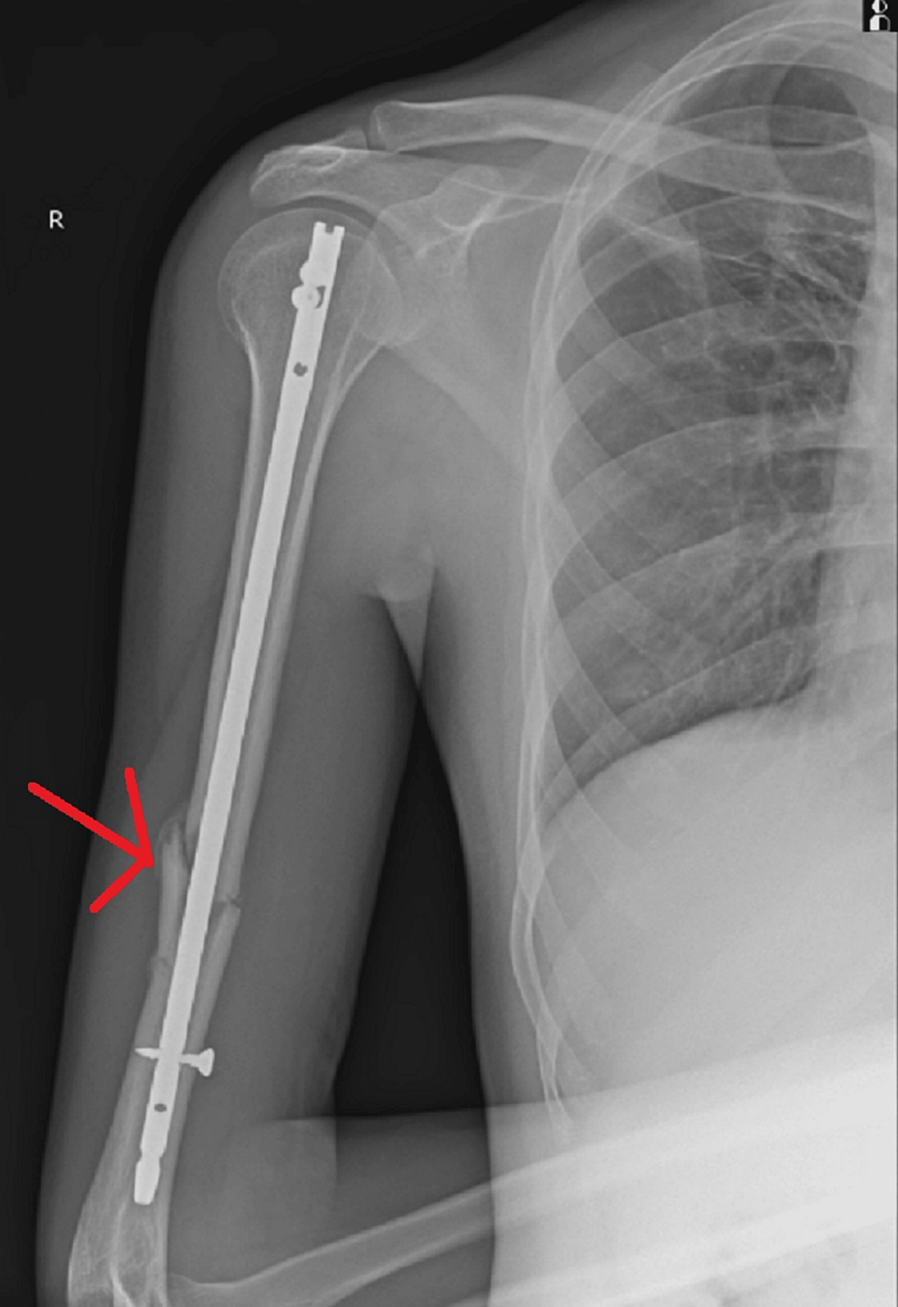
Post-one-month radiograph showing CRIF with intramedullary nailing for mid-shaft humerus fracture on the right side CRIF - closed reduction and internal fixation
